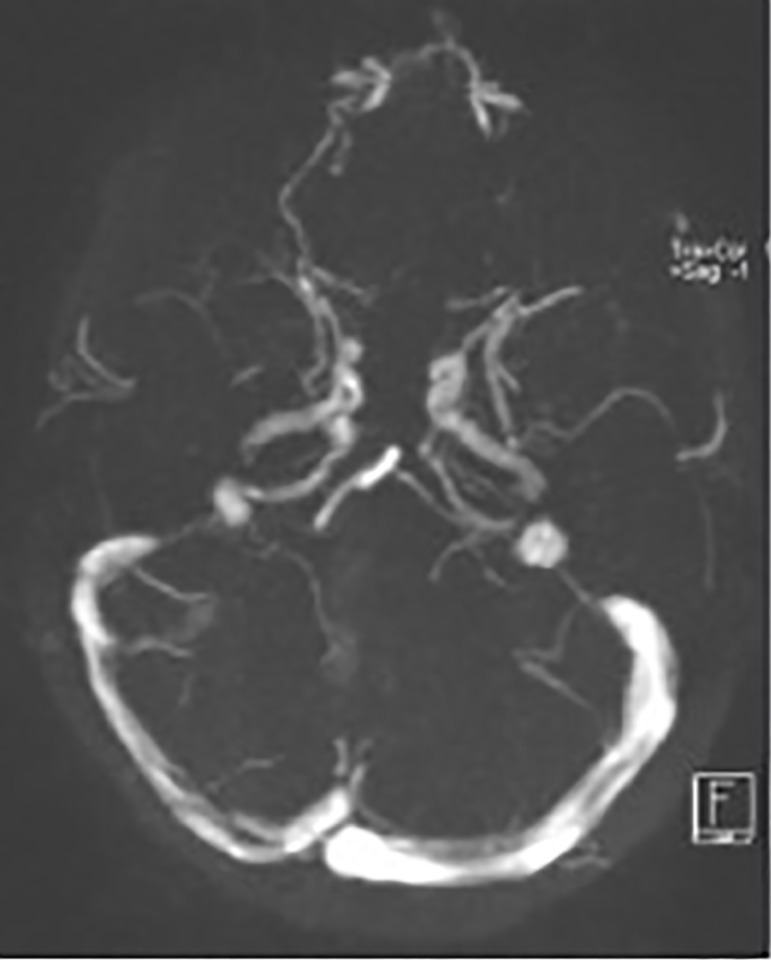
Baseline head MRI. Source: Electronic Patient Chart.
